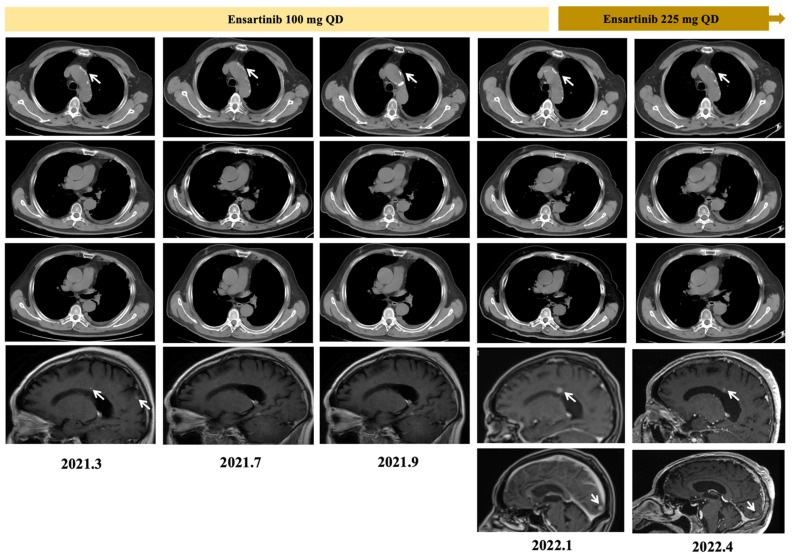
Thoracic computed tomography (CT) and brain MRI images recorded treatment-related changes of the patient under ensartinib.
